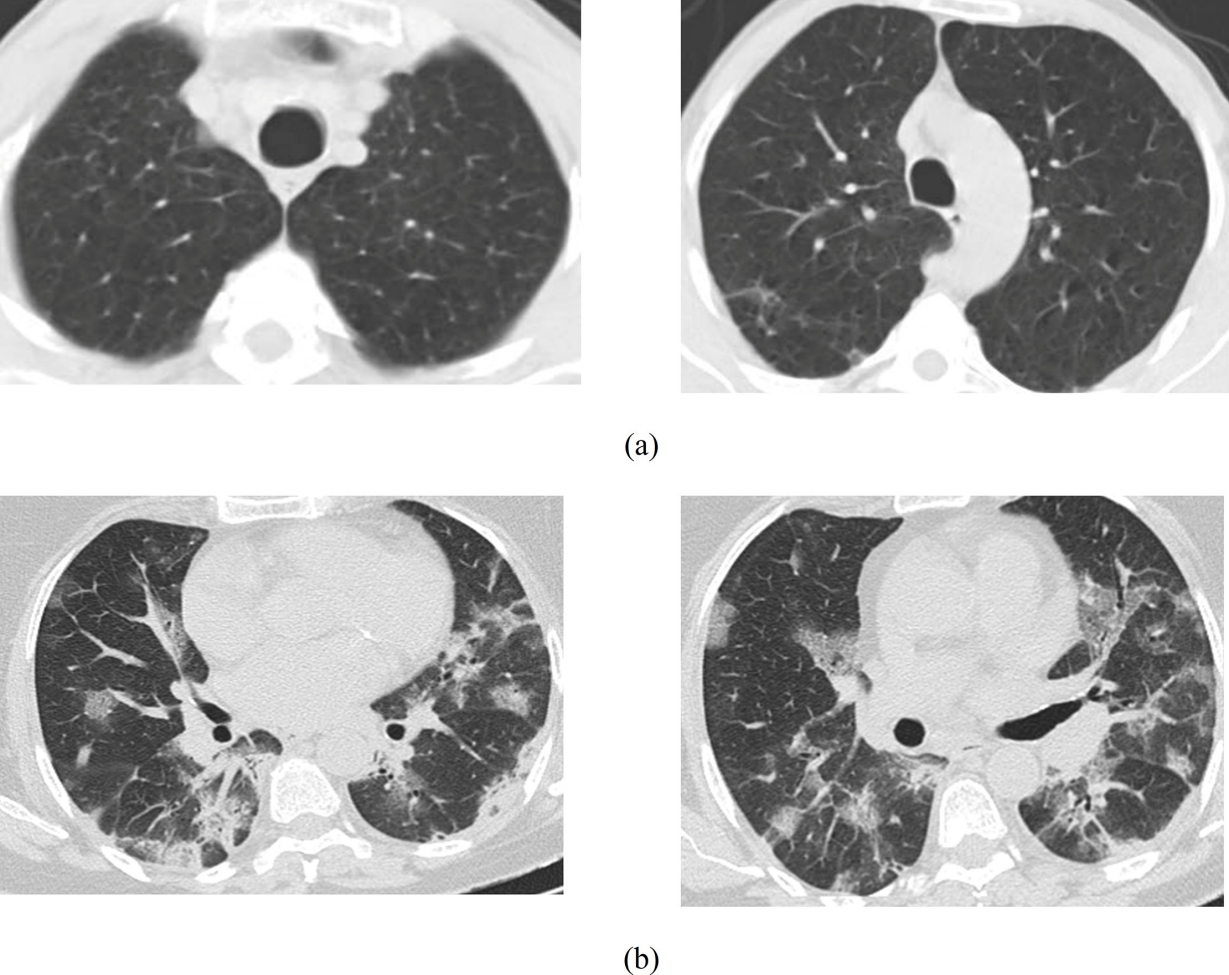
Sample of the (a) non-COVID-19 and (b) COVID-19 CT scan dataset [53].
